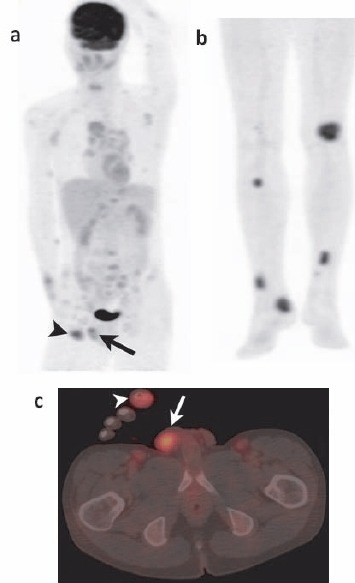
Maximum intensity projection (MIP) image of FDG-PET/CT) (a, b). Abnormal FDG accumulation of multiple lymph nodes and skeletal lesions concordant with bone scintigraphy. FDG-PET/CT showed abnormal FDG accumulation on right testis (arrow) and right forefinger (arrow head) (c). Bone destruction of the distal phalanx of the right forefinger was found.
